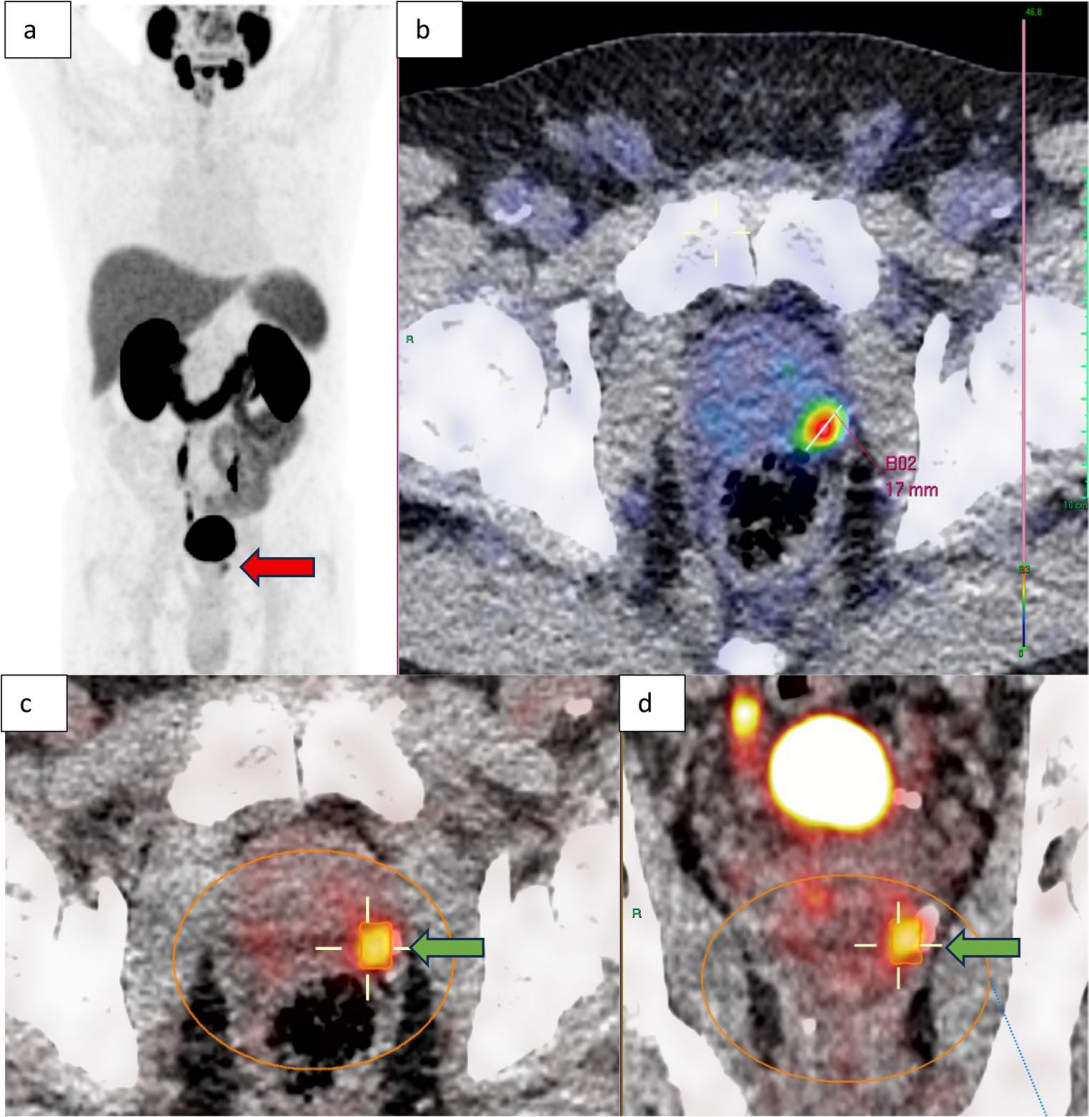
Staging [^18^F]DCPyL PET maximum intensity projection showing focal prostate tumour activity (red arrow) (a). Fused axial PET/CT images using a gradual “Rainbow” color look-up table and scaled to tumour SUVmax (in line with methods described by Brauchli et al. and Hvittfeldt et al.) showing LCC measurement on the prostate tumour (PET) – capsule (CT) interface (b). Fused axial PET/CT images using standard clinical color look-up table (axial view, c; coronal view, d) showing prostate tumour delineation (green arrow) within a VOI using a SUV threshold of 4, allowing measurement of SUVmax, SUVpeak, PSMA-vol and PSMA-TL
